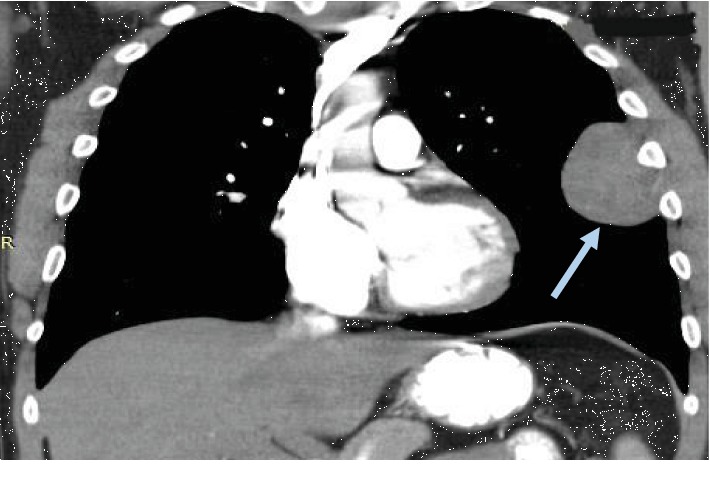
Solitary fibrous tumor of the left pleura (grey arrow). Computed tomography, thorax. Coronal view.
